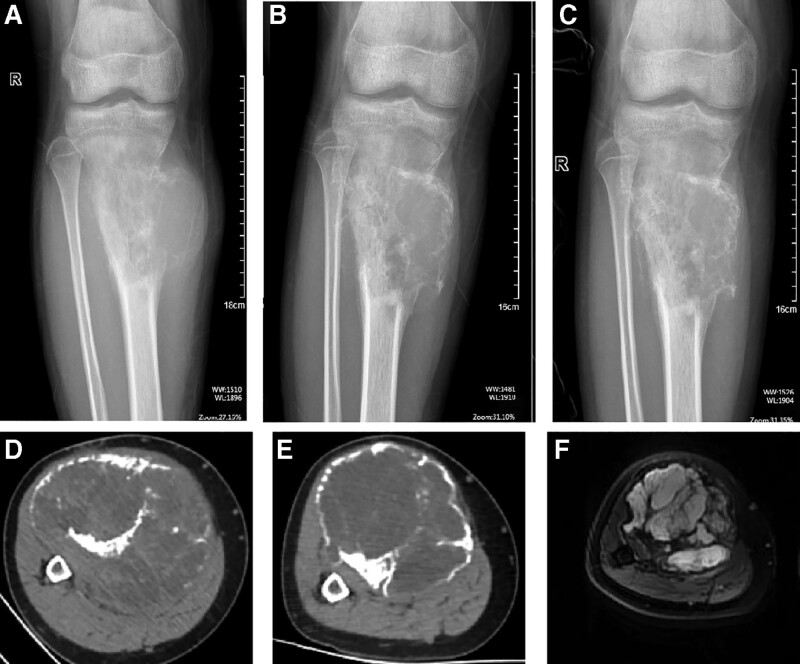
Plain radiograph: Before chemotherapy, the density of the proximal right tibia decreased, with a huge soft tissue mass surrounding it and an unclear boundary (A). The soft tissue volume was significantly reduced and the calcification was obvious after the addition of denosumab (B and C). Computed tomography images before (D) and 3 times after the administration of denosumab combined with chemotherapy (E).
